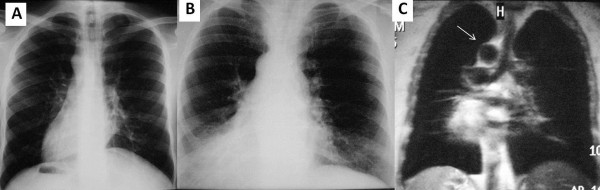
Thoracic MRI and Chest radiograph images Chest radiographs showing dextrocardia with RSAA (6A, 6B). Thoracic MRI showed that there was no tracheal compression due to RSAA (6C, white arrow).
